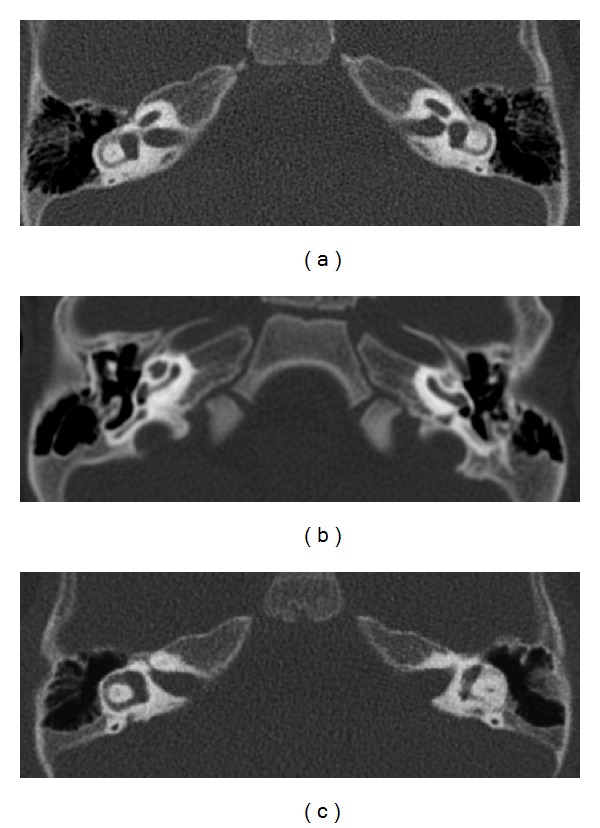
High-resolution computed tomography of the inner-ear structures of the patients: (a) II-3 and (b) II-5. There is no evidence of malformations of the cochlea or the petrous bone; (c) control image.
